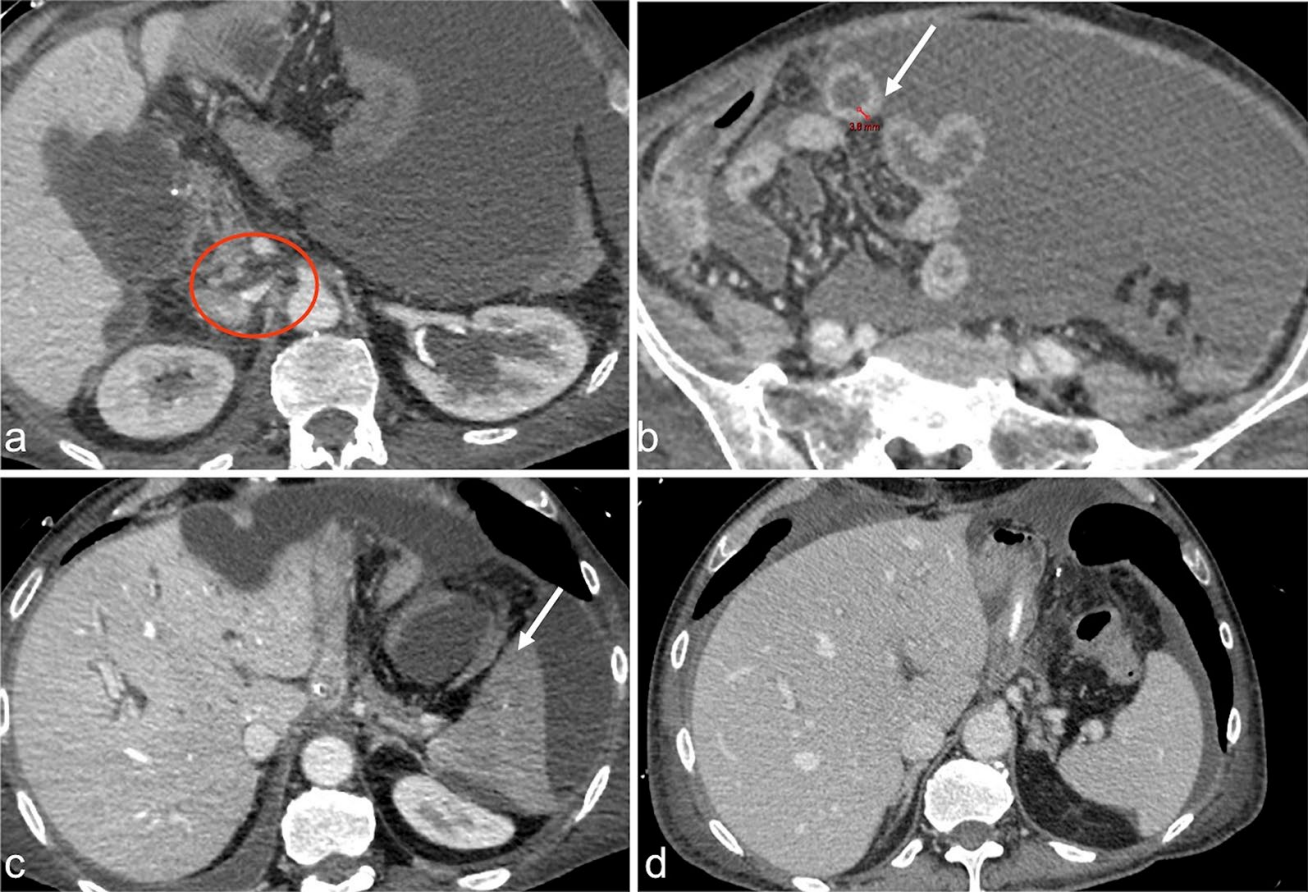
Contrast-enhanced CT in the portal venous phase, in the axial view, showing a 70-year-old male with sepsis due to gastric cancer surgery complications (qSOFA 3). In this patient, multiple CT signs of sepsis can be observed, including inferior vena cava halo sign (a, red circle), thickened bowel wall (b, white arrow), reduced enhancement, and spleen volume (c, white arrow). Figure d shows a previous CT examination of the same patient for comparison
